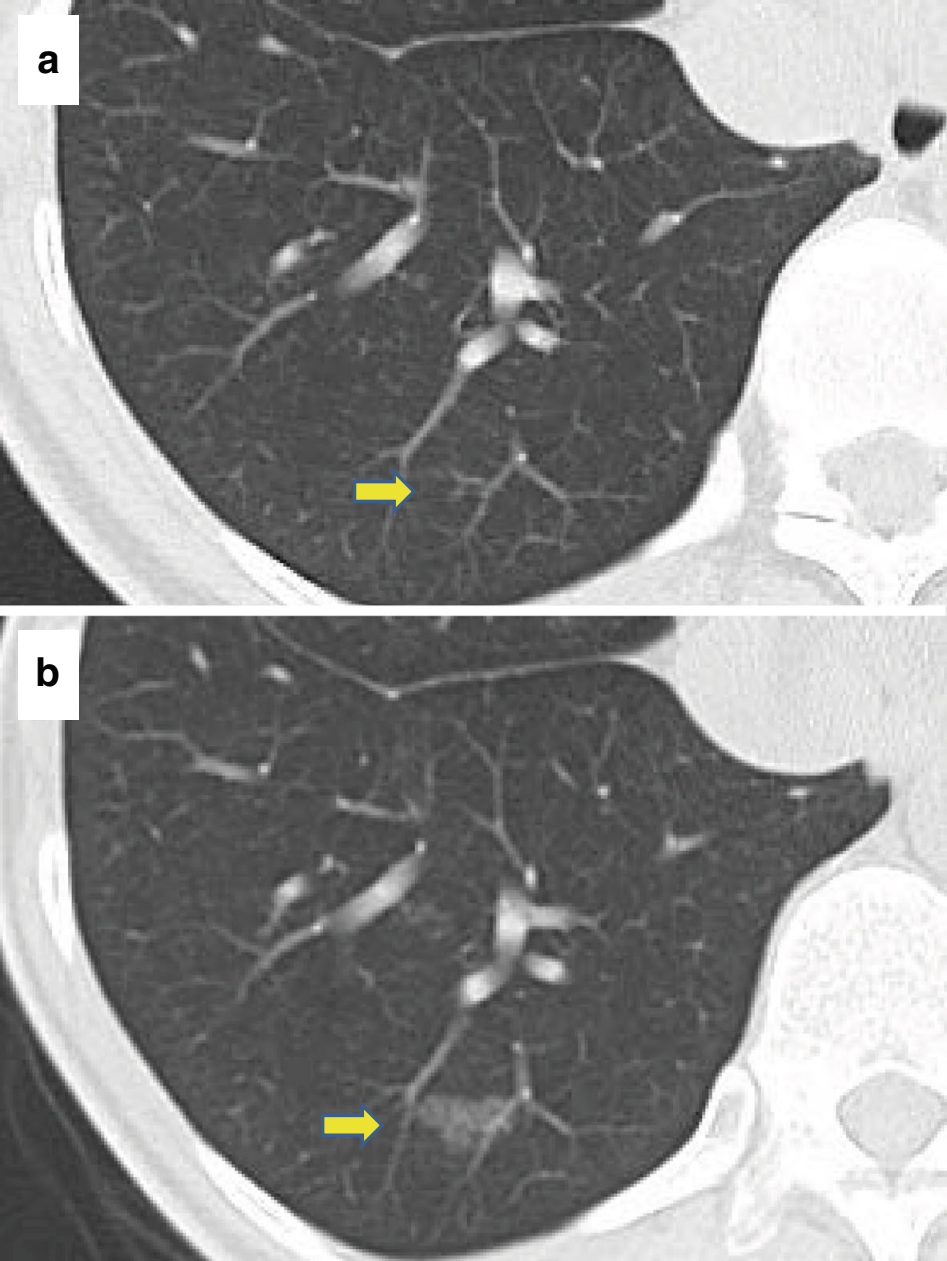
Chest computed tomography in 2012 revealed a very small shadow with ground glass opacity (*arrow*) that increased in size over time. a in 2012 (b) in 2015
